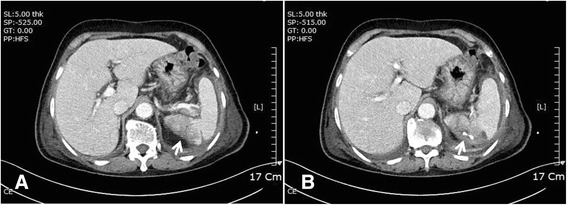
Computed tomography shows splenic infarction (a, *white arrow*) and abscess with percutaneous drainage (b, *white arrow*)
